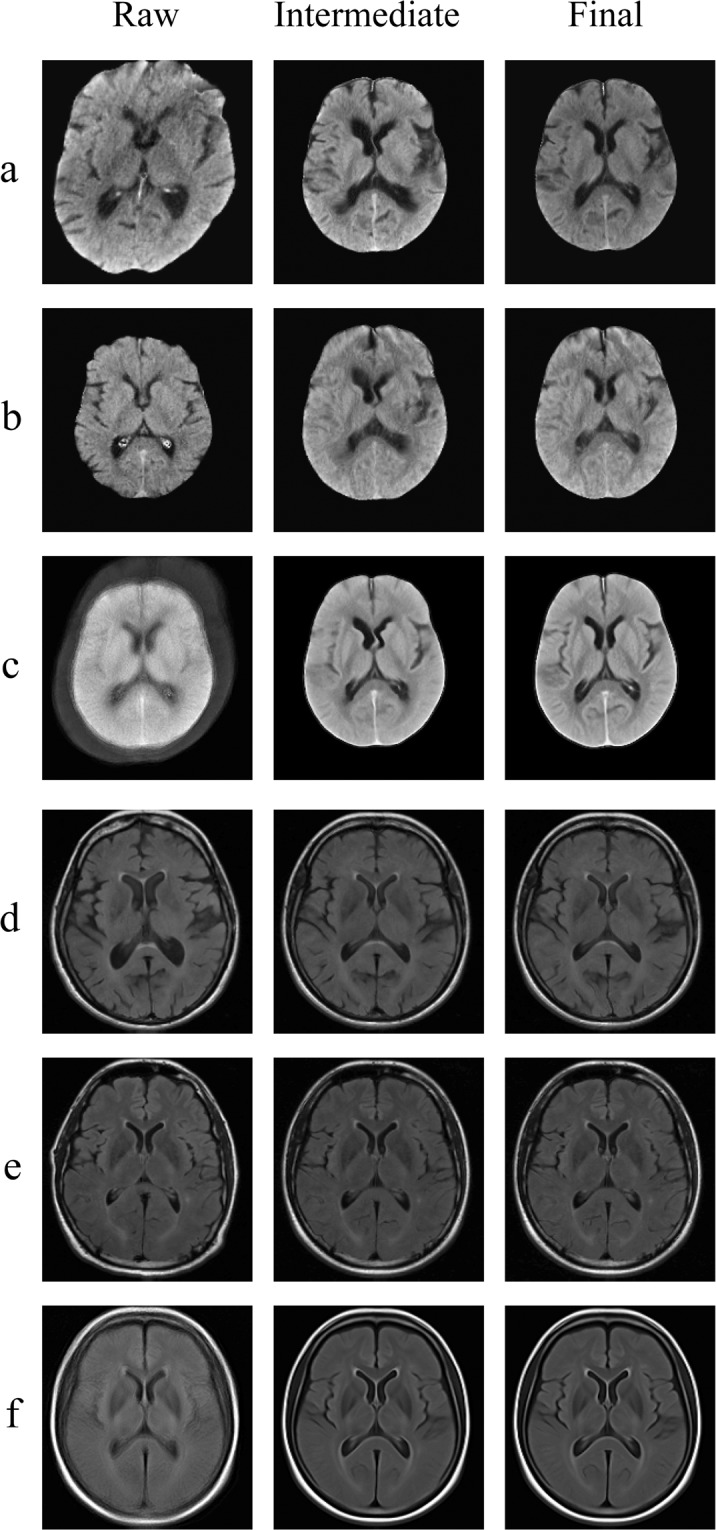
Atlas generation. (a) sample NCCT with large ventricles; (b) sample NCCT with small ventricles; (c) MIPLAB-NCCT atlas; (d) sample FLAIR with large ventricles; (e) sample FLAIR with small ventricles; (f) MIPLAB-FLAIR atlas.
